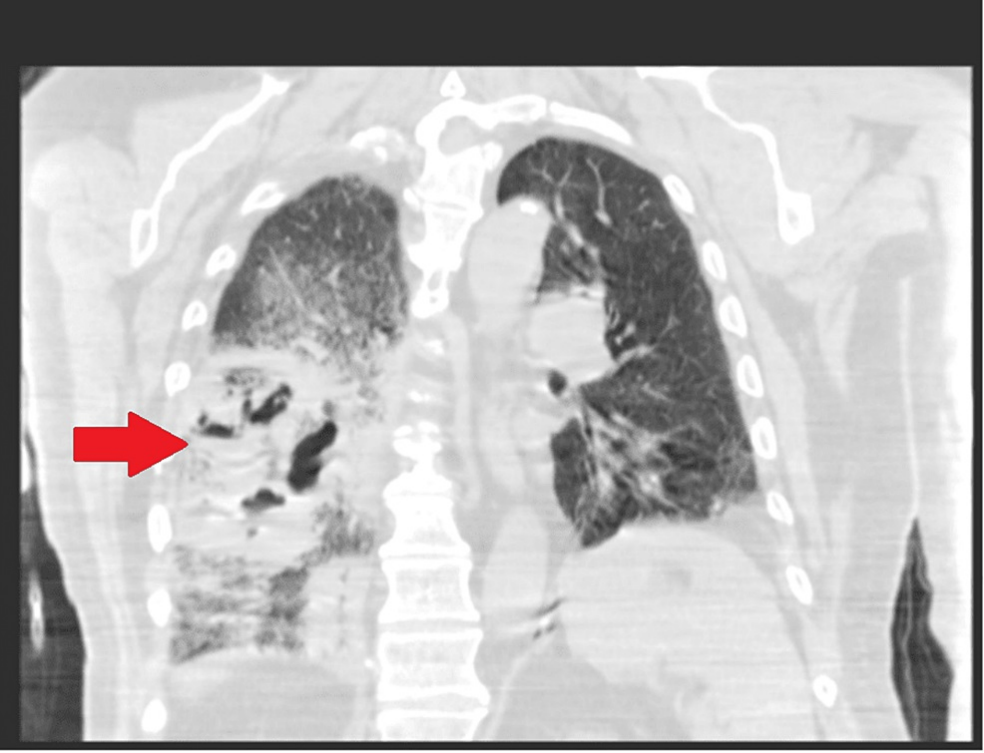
CT chest coronal view showing right lower lobe cavitary lesion.
